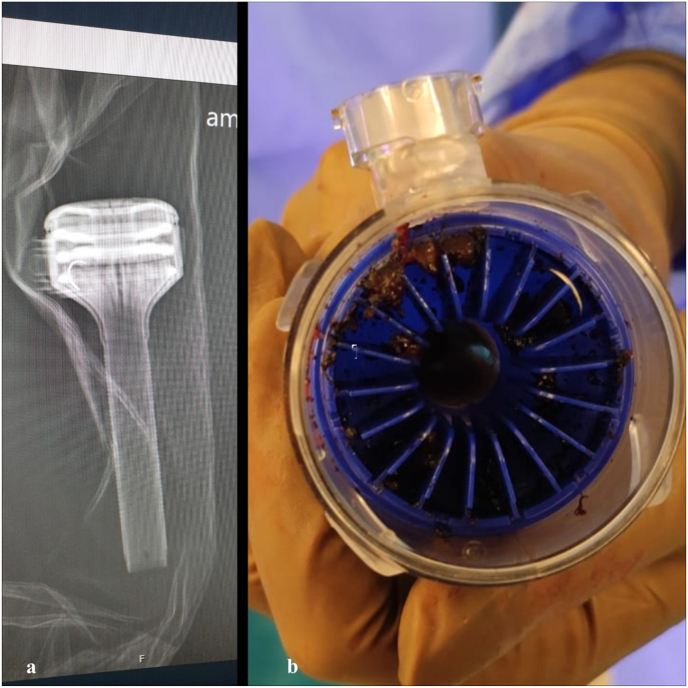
A. The appearance of a needle stuck inside an Airseal trocar under x-ray radiography. 1b. The image of a needle stuck in the air channels of a disassembled Airseal trocar.
